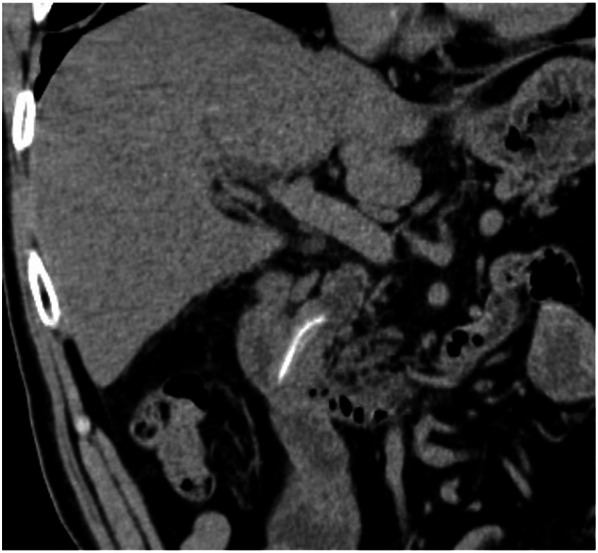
The coronal plane computed tomography (CT) image showing a common bile duct (CBD) stone along the length of the CBD, with a needle-shaped calcification density at the center
